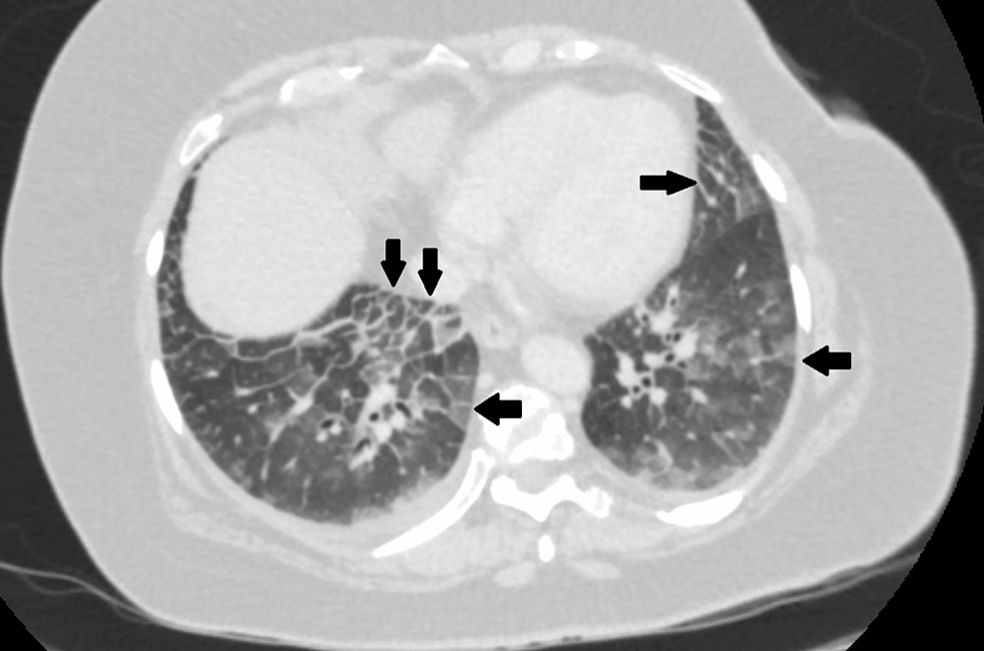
CT abdomen with contrast (case 2) - patchy crazy paving pattern opacification (arrows) seen on lung window at the lung bases. Crazy paving: ground-glass opacification with superimposed interlobular and intralobular septal thickening. CT: computed tomography
